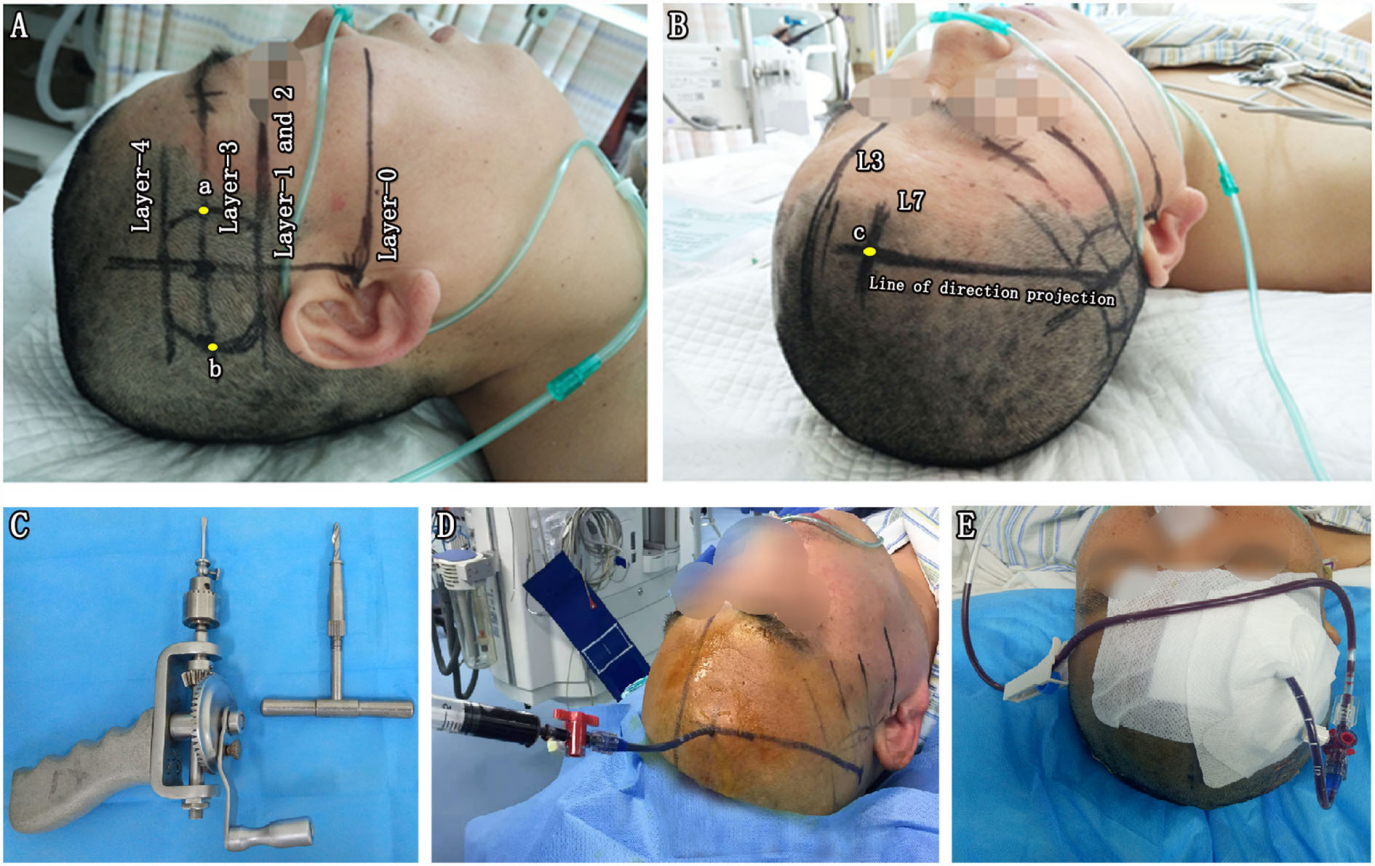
Parameter reproduction on a patient's head and free-hand catheter technique. (A) Reproducing the actual baseline plane of the CT scan on the patient's head and outlining the sagittal projection of the hematoma border. With the help of a triangle ruler, two parallel baselines were drawn passing through the external auditory canal (Layer-0) and eye's lenses (Layer-1) with the distance of D1. Next, the CT scan axis was drawn through the external auditory. The projection lines of the bottommost (Layer-2), largest (Layer-3), and uppermost (Layer-4) layers of BGH were depicted on the ipsilateral temporal skin, according to the distance (D2, D3, and D4) to Layer-0. Layer-1 and Layer-2 exactly coincided in this case. On the projection line of Layer-3, the projection points of the anterior (point a) and posterior (point b) borders of the hematoma were marked according to D5 and D6. (B) The midsagittal line L3 and paramedian sagittal line L7 were drawn with the reference of D7. The puncture point (point c) was located at the forehead area between 2 cm above eyebrow arch and coronal suture away from the vascular and functional distribution of brain tissue. A marking line of the puncture direction was drawn from point c to the center of the temporal skin projection area of the BGH. (C) A hand drill and a reamer. (D) Aspiration with a 5-mL syringe. (E) Hematoma drainage. BGH, basal ganglia hematoma.
